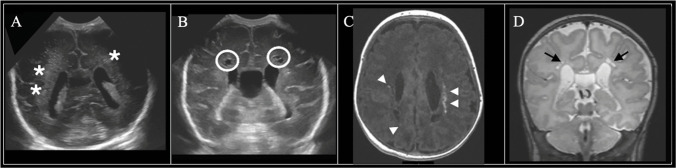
Sequential neuroimaging in a male infant born at 29 weeks and 5 days. (A) 7-DOL coronal-plane head ultrasound demonstrated focal areas of abnormal echogenicity greater than or equal to choroid plexus (*asterisk*, Miller score 3); (B) 30-DOL coronal-plane head ultrasound showed cavitary cystic lesions in the white matter (*white circles*, Miller score 5). Term-equivalent MRI demonstrated (C) greater than 6 foci of increased signal intensity in the white matter on axial T1-weighted imaging (*white arrowheads*) and (D) extensive periventricular cysts (*black arrow*) on axial T2-weighted imaging, with ventriculomegaly and decreased white matter volume (Martinez-Biarge score 3)
